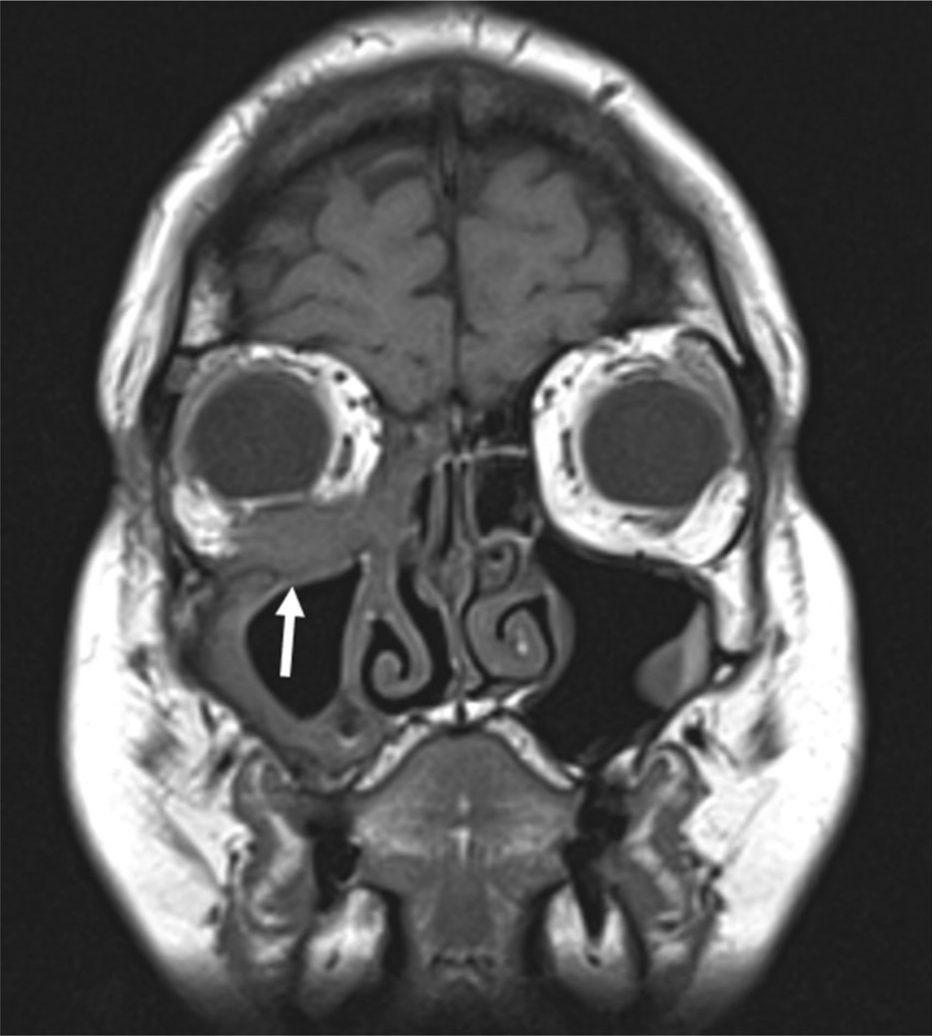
Coronal T1 weighted MRI orbit in a 49‐year‐old female patient with GPA showing a soft tissue mass with destruction of the floor of the right orbit extending into the right orbital inferior extraconal space that was inseparable from the adjacent inferior rectus (arrow). The patient was later diagnosed with GPA when biopsy of the right maxillary uncinate and ethmoid sinus showed necrotising granulomatous inflammation.
